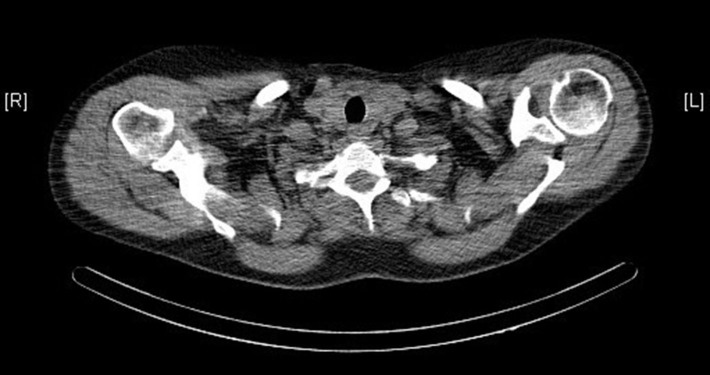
CT scan of the neck showing a normal thyroid gland.
